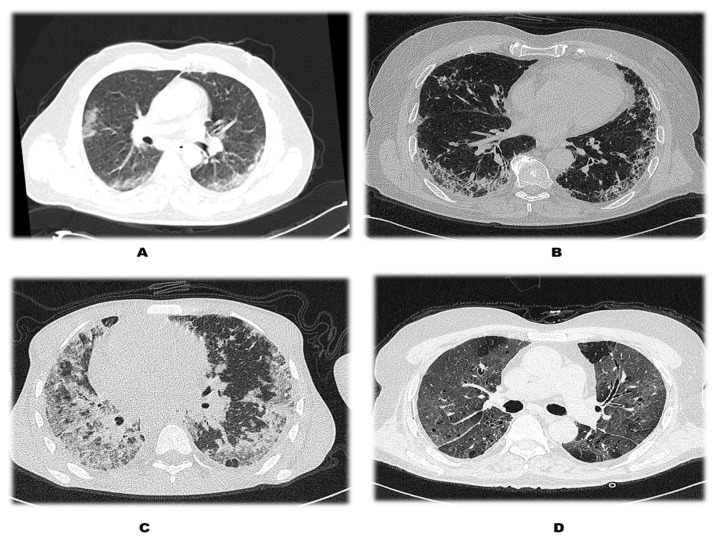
Pictorial representation of the severity of COVID-19 axial HRCT chest cuts with lung window demonstrating severity of disease: (A) Left upper—mild disease, (B) right upper—moderate disease, (C,D) severe disease.
